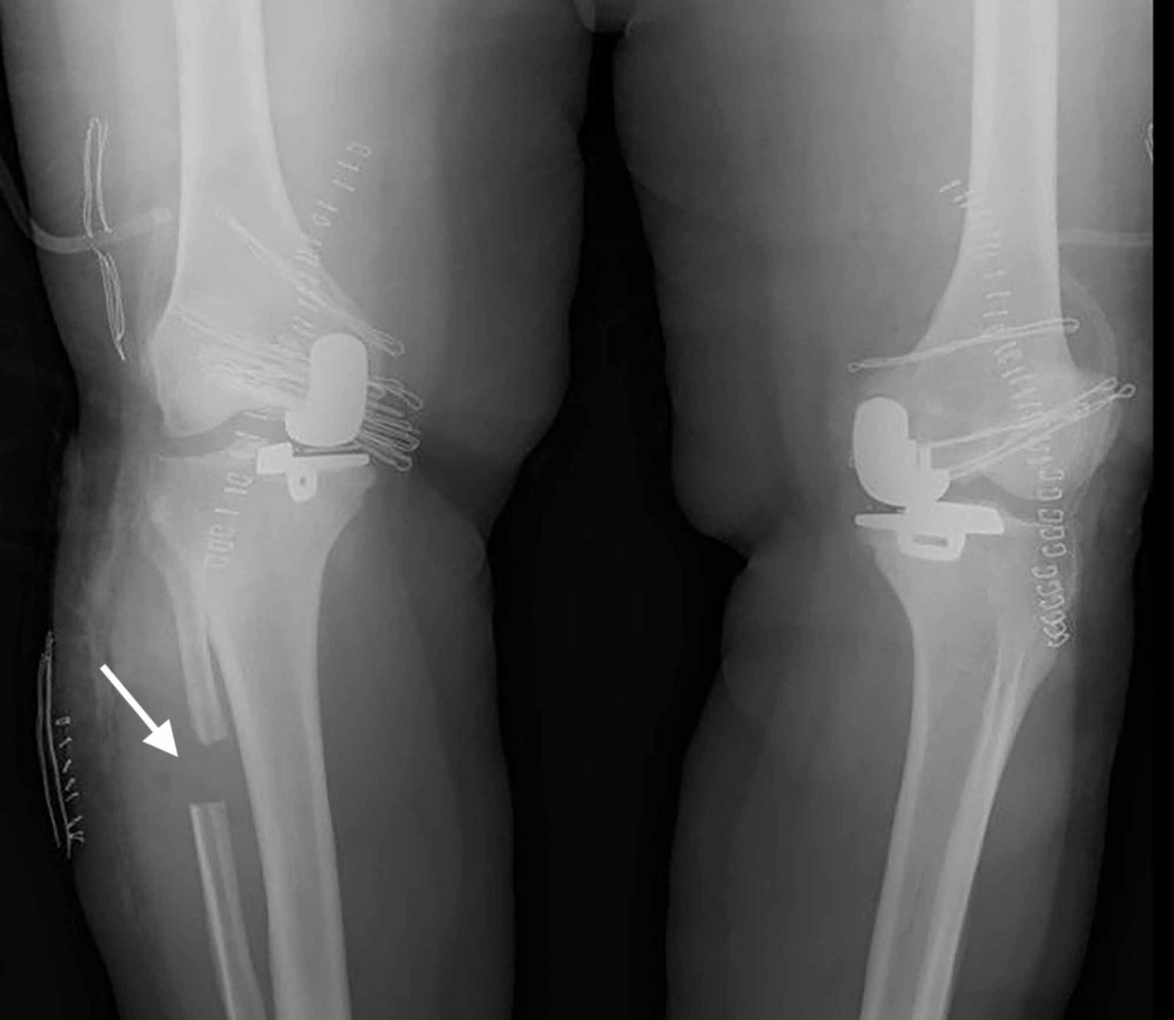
Postoperative antero-posterior (AP) radiograph of both knees; arrow indicates the proximal fibular osteotomy site. Note the staples of the short incision on lateral aspect
